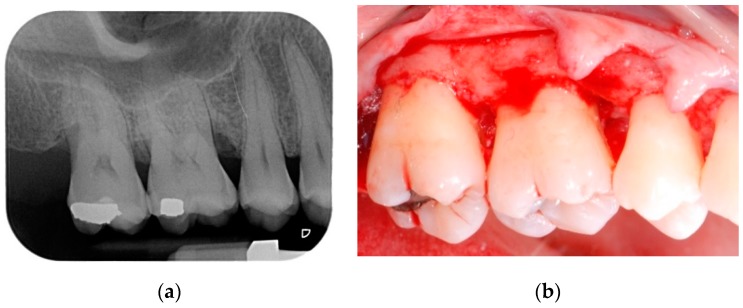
(a) Pre-operative periapical radiograph; (b) A full thickness flap was elevated in order to get access to the root and the periodontal intrabony defect.
